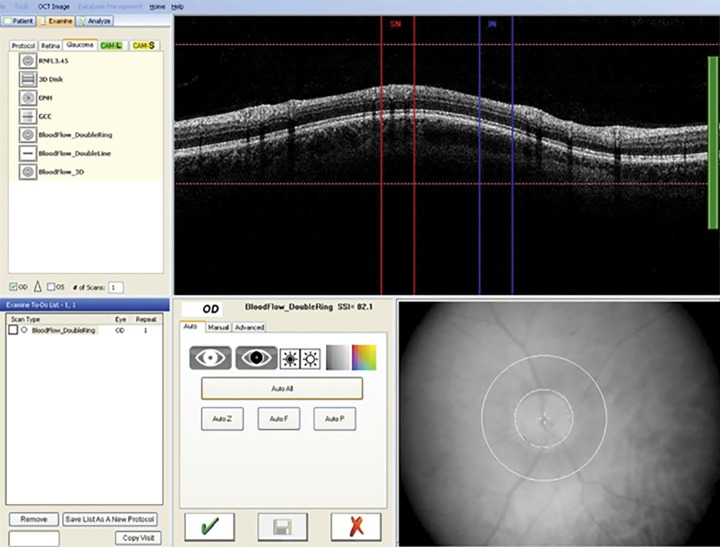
Double circular Doppler scan (two concentric rings around the optic nerve)
